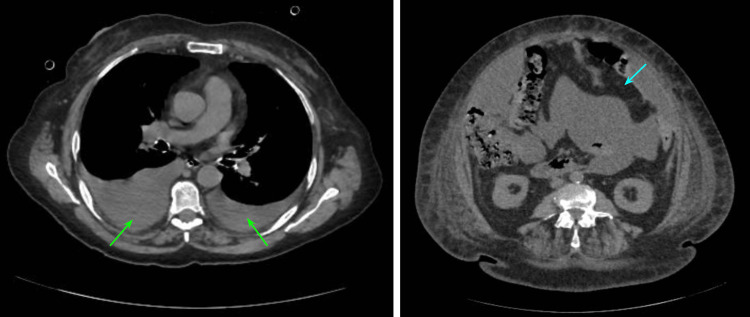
Postoperative abdominal CT scan. The CT scan shows bilateral pleural effusions (green arrows) and abundant ascites fluid (blue arrows).
